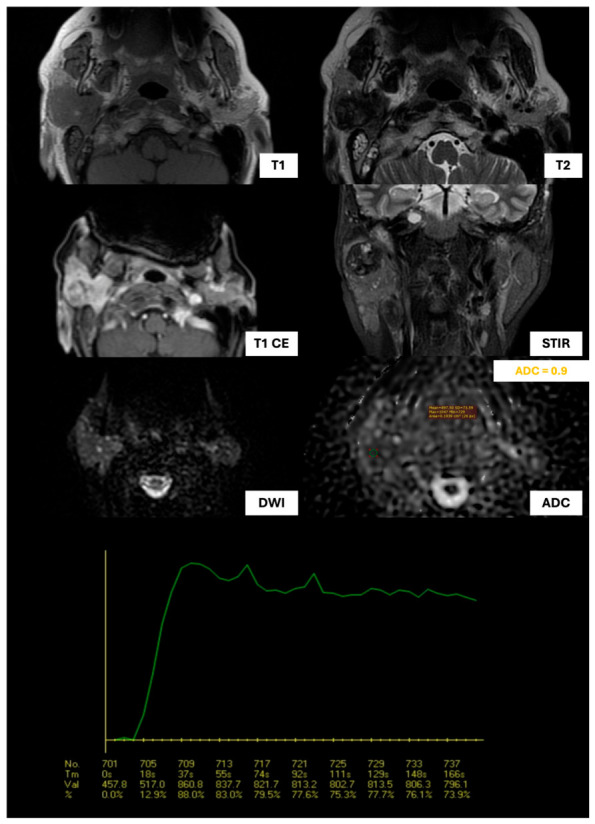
Salivary duct carcinoma of the right parotid gland, demonstrating a type C enhancement curve on DCE-MRI. The green circle indicates the region of interest (ROI) placed within the tumor in order to measure the ADC value.
